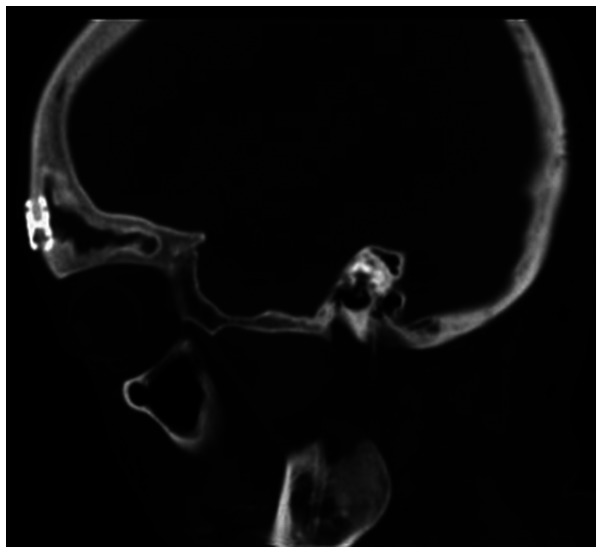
Frontal sinus sagittal view CT at 1 year postsurgery demonstrated that the titanium bone fixation set was in place and that there was no space-occupying lesion in the frontal sinus cavity.
